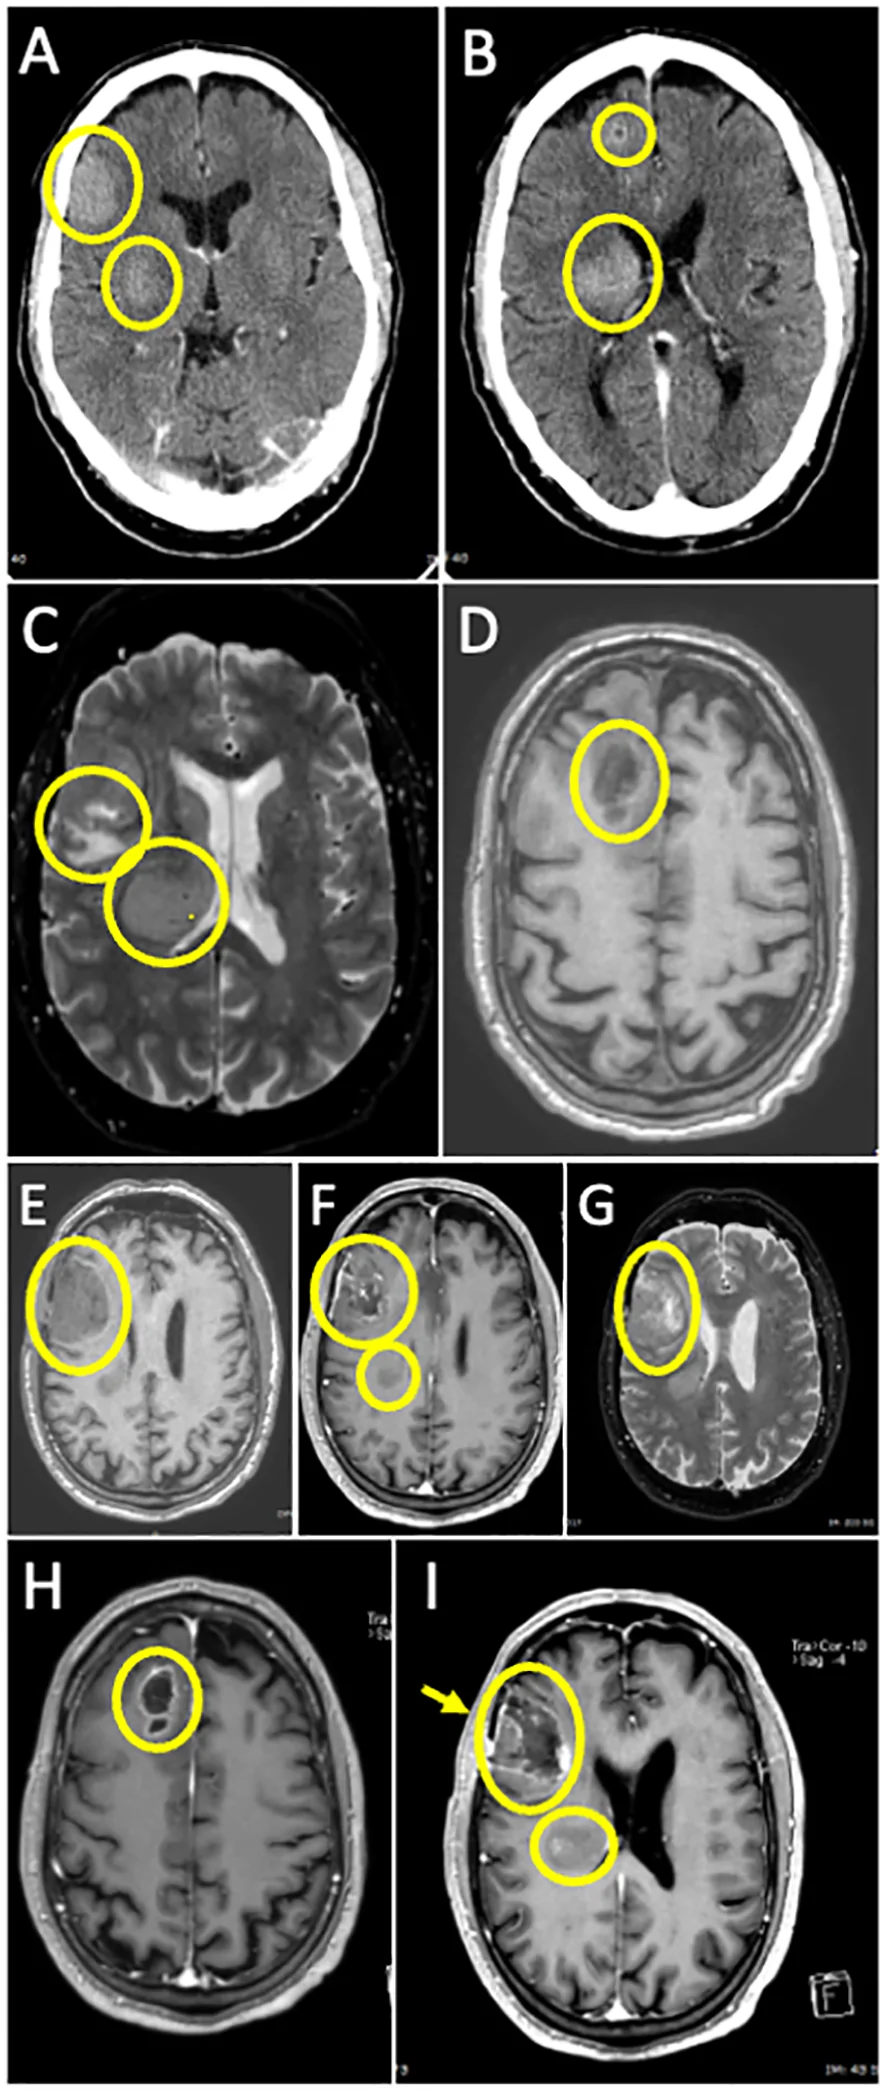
Preoperative computed tomography (CT) scans (A, B) and magnetic resonance imaging (MRI) (C-H) demonstrating multiple intra-axial lesions within the right cerebral hemisphere, consistent with multifocal disease. Yellow circles indicate the lesions. The largest lesion was located in the right frontal cortex, measuring 28 × 18 mm (E-G). An additional lesion measuring 25 × 23 mm was identified in the deep periventricular region with involvement of the right thalamus (C). Three smaller intra-axial nodules, each measuring approximately 10 mm, were also identified in the right hemisphere (D, H). Postoperative MRI (I) shows the resection cavity, with the yellow arrow indicating the site of the resected right frontal lesion.
